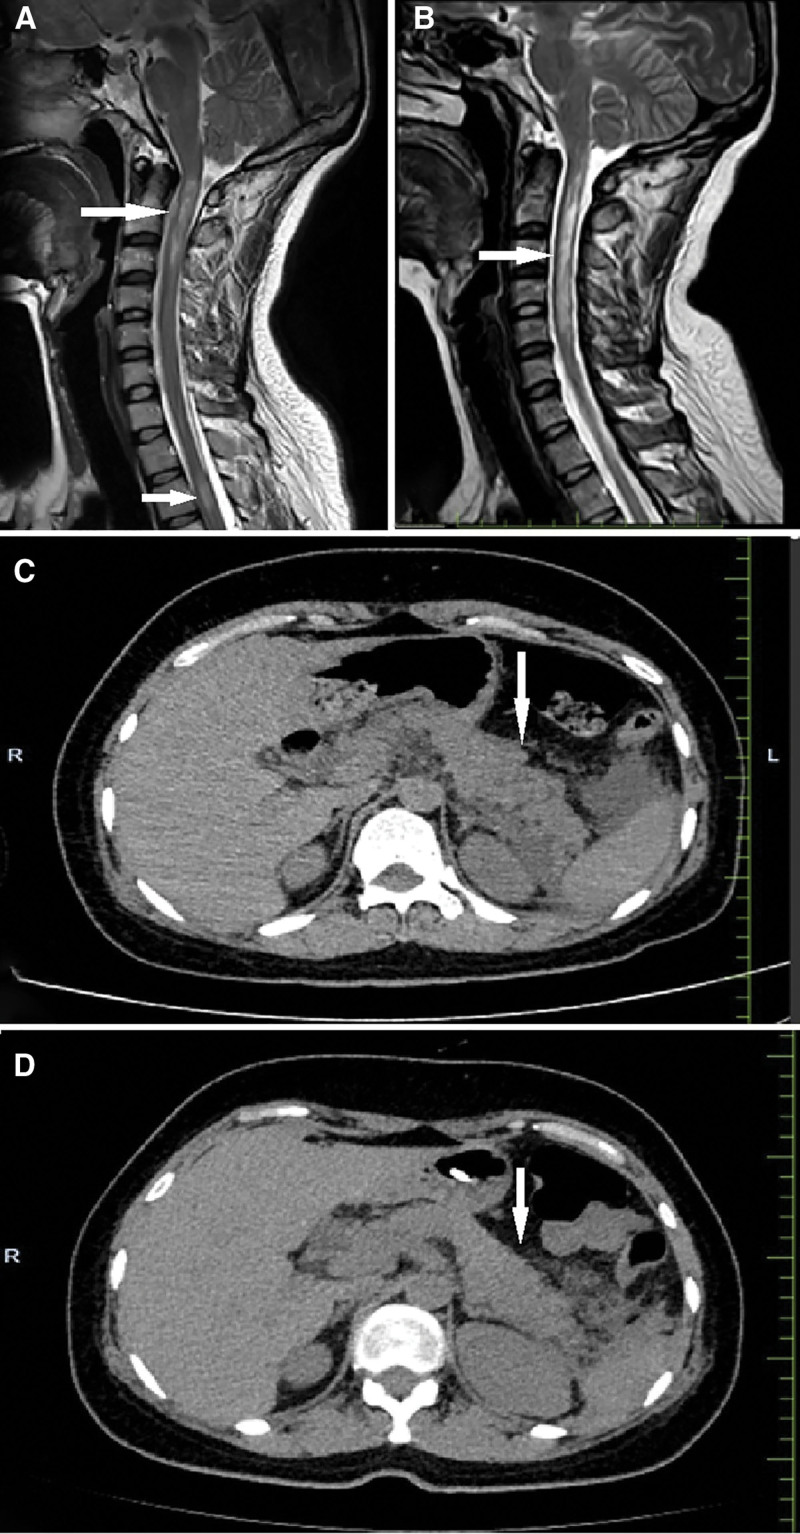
Spinal MRI scans on the T2-weighted sequence showed 2 hyperintense lesions at C2/C4 and T2 vertebral levels (arrow) at the initial onset (A) and extending from C2 to C6 vertebral levels (arrow) at the recurrence of the disease (B). Abdominal CT scan showed typical signs of AP (arrow), including enlargement of the pancreatic body and tail with irregular contours, blurred surrounding fat spaces, and fluid accumulation (C), and significant improvement in pancreatitis imaging findings after treatment (D). AP = acute pancreatitis, CT = computed tomography, MRI = magnetic resonance imaging.
